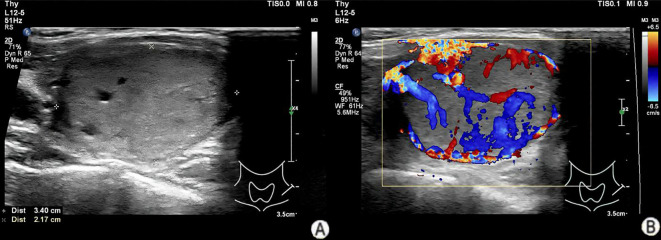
Thyroid ultrasound image: A hypoechoic nodule measuring approximately 3.4 cm × 2.2 cm × 2.5 cm is visible in the middle-lower portion of the left thyroid lobe. It exhibits a regular shape, clear margins, a length-to-width ratio <1, and heterogeneous echogenicity (A). Color Doppler flow imaging (CDFI) demonstrates abundant internal blood flow signals (B).
